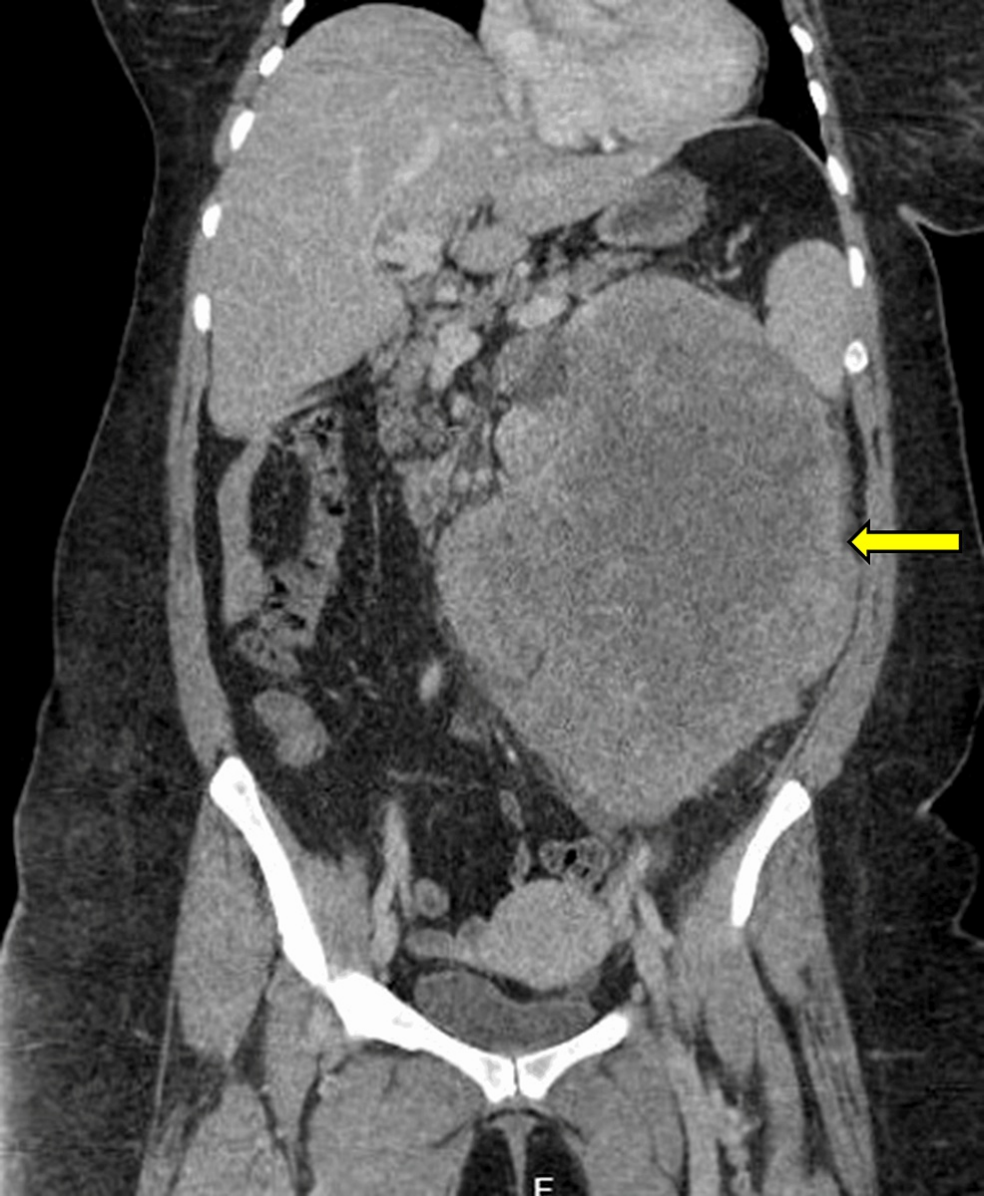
Coronal CT image of primary Ewing sarcoma of the left kidney measuring 25 cm x 18 cm x 18 cm (arrow) CT: computed tomography
